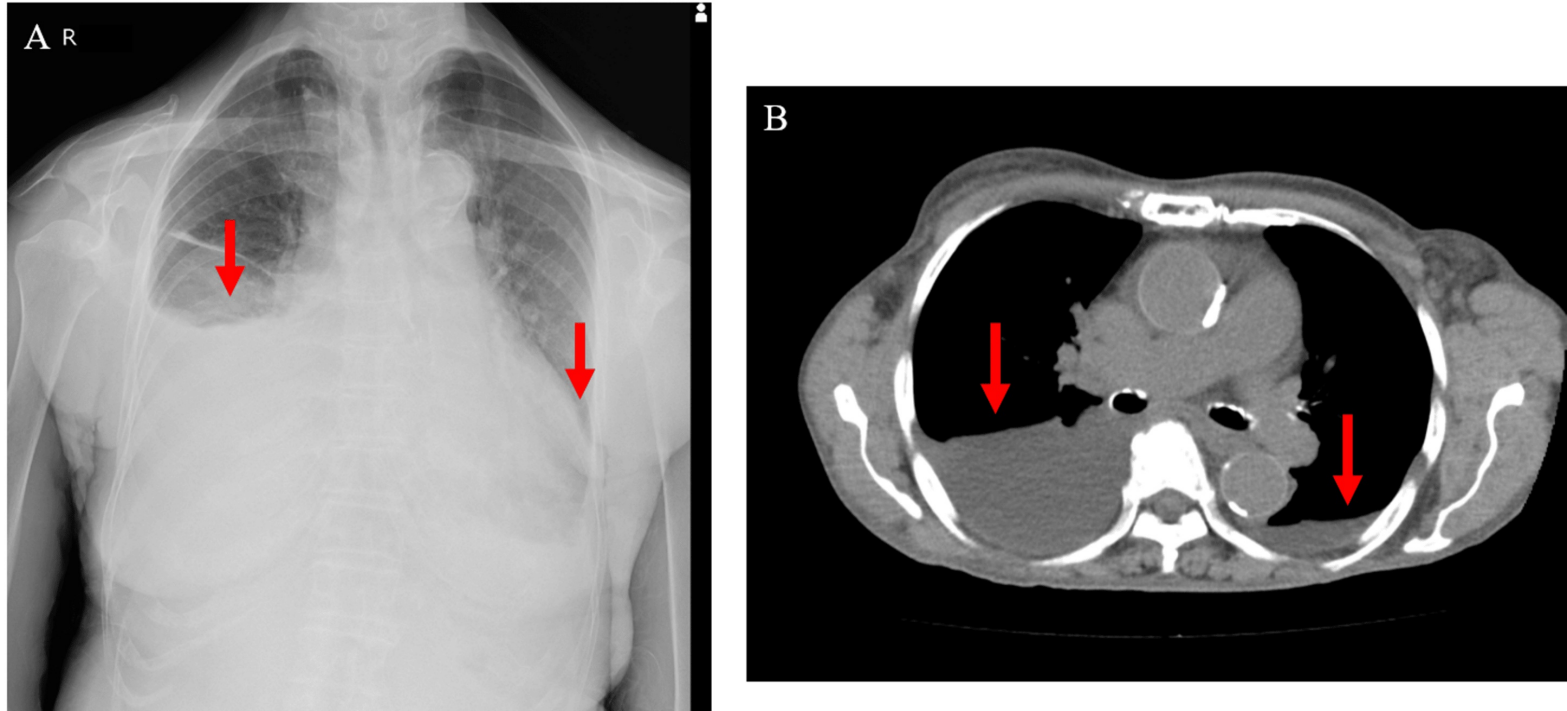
Pleural effusion (red arrow) on chest X-ray (A) and chest CT scan (B) CT: computed tomography
